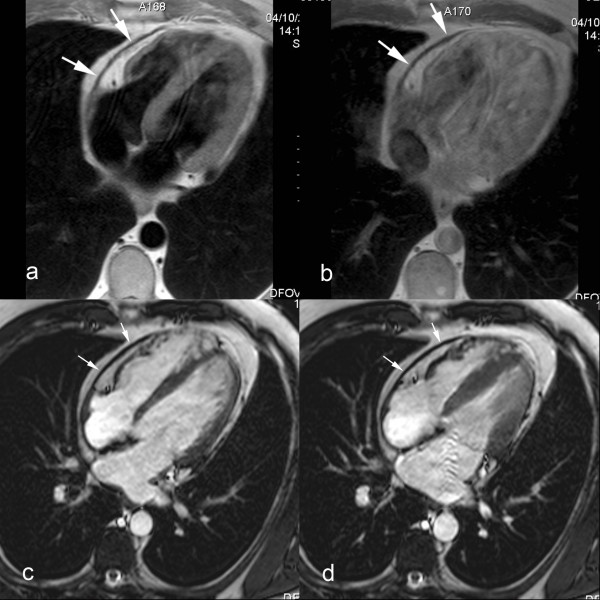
Calcified constrictive pericarditis, axial T1-weighted spin-echo CMR before (a) and after (b) administration of gadolinium-chelates, cine CMR at end diastole (c) and end systole (d) in the same imaging plane. A mildly thickened, hypointense appearing pericardium is visible over the right heart (arrows). No enhancement of the thickened pericardium after gadolinium administration (b). The thickened part of the pericardium has a rigid, immobile appearance, while the underlying myocardium exhibits a normal contraction pattern. The dynamic viewing mode allows a better appreciation of the differences in motion between the rigid pericardium and underlying myocardium.
